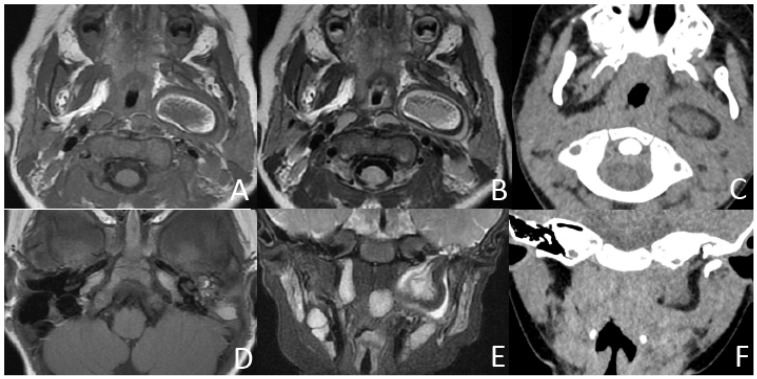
MRI (conducted at 11 months) and CT (conducted at 2 years and 7 months) of a girl who failed newborn hearing screening of the left ear, presenting with left otopyorrhea from 2 years old. (A,B) Axial T1-weighted, T2-weighted MRI show a multilayered circumferential mass in the left parapharyngeal space that is soft tissue, lipid, fibrous capsule, and soft tissue intense from inside to outside. (C) Axial CT shows a 3-layered circumferential mass that is soft tissue dense, fat dense, and soft tissue dense from inside to outside. (D) Axial T1-weighted MRI shows an irregular hyperintense and isointense mass in the left middle ear. (E,F) Coronal T2W-SPIR MRI and CT show the pear-like multilayered circumferential mass in the left multilayered circumferential mass with the pedicle extending to the left middle ear and the bone defect of the inferior wall of the external auditory canal and mastoid process.
